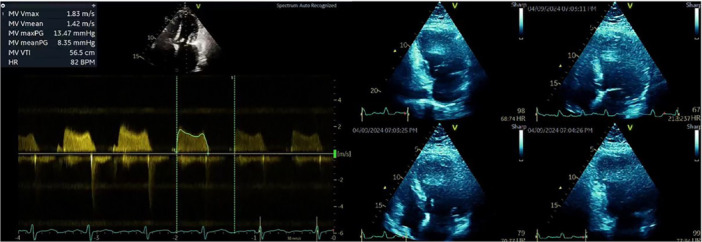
A postprocedure transthoracic echocardiogram. The MV mean gradient of 8 mmHg and improving the valve area to 1.5 cm^2^. [Color figure can be viewed at wileyonlinelibrary.com]
